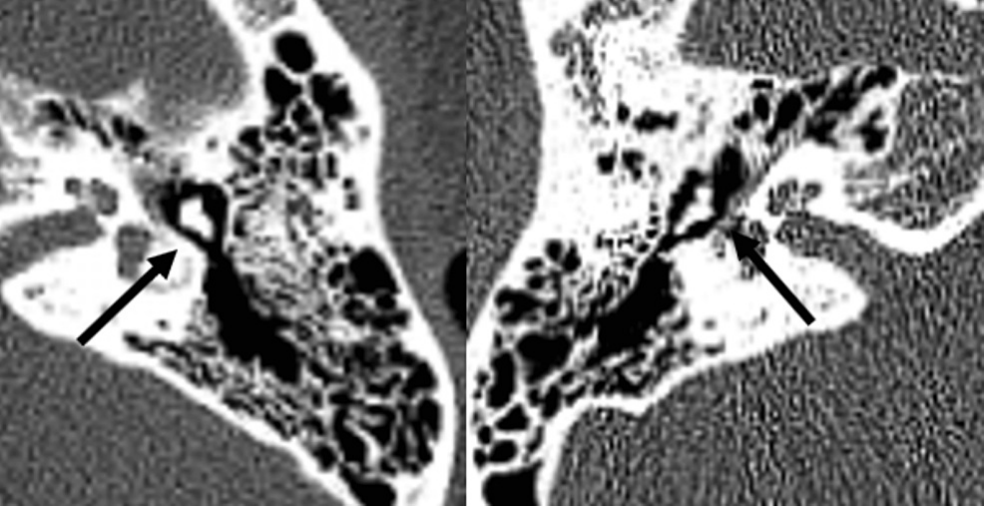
Axial thin-cut bone window CT scan of the temporal bone showing a bilateral tympanic segment of facial canal wall dehiscence (black arrows).
